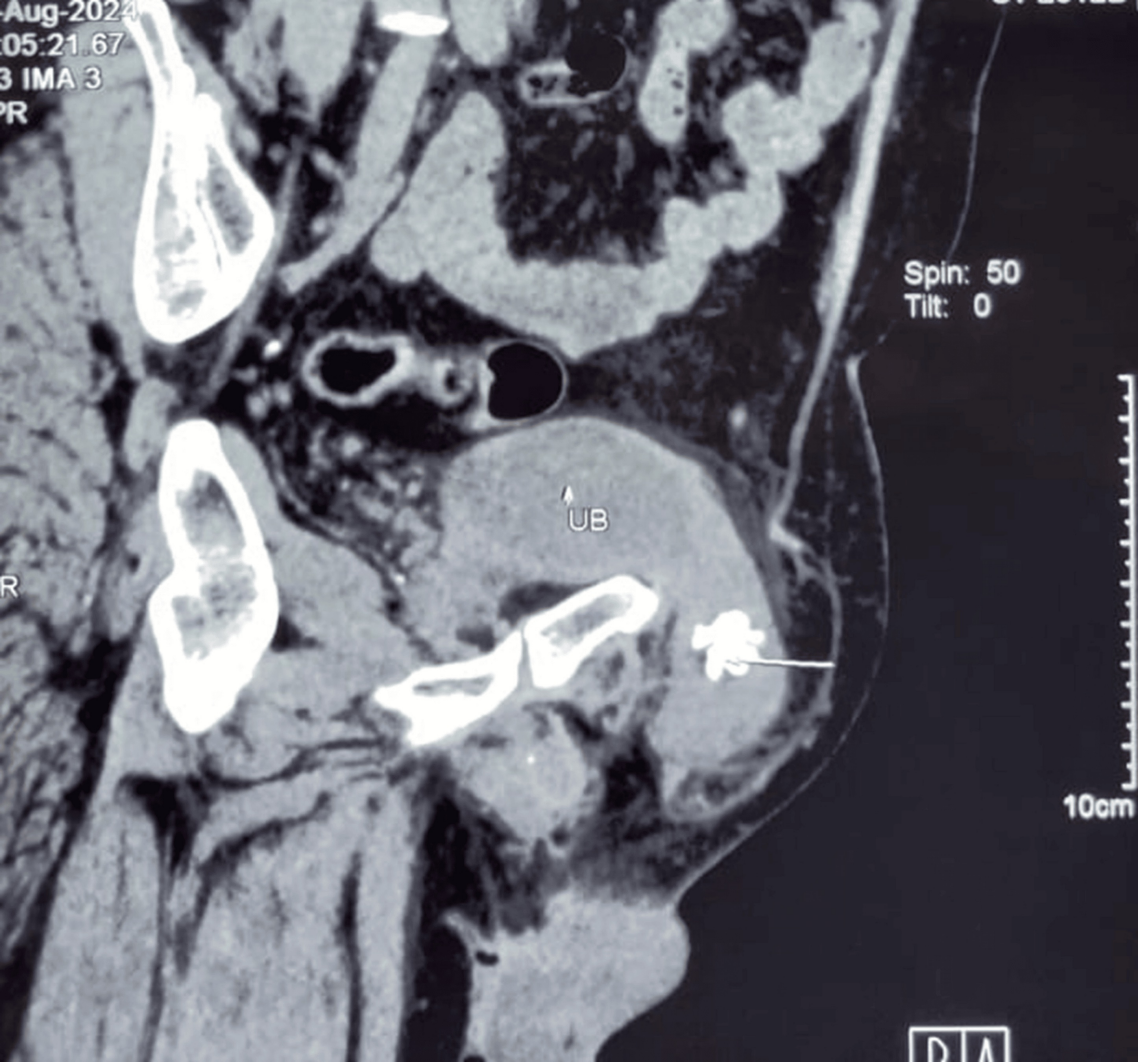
Computed tomography showing herniation of the urinary bladder into the left inguinal canal with an intravesical calculus (small white arrow)
